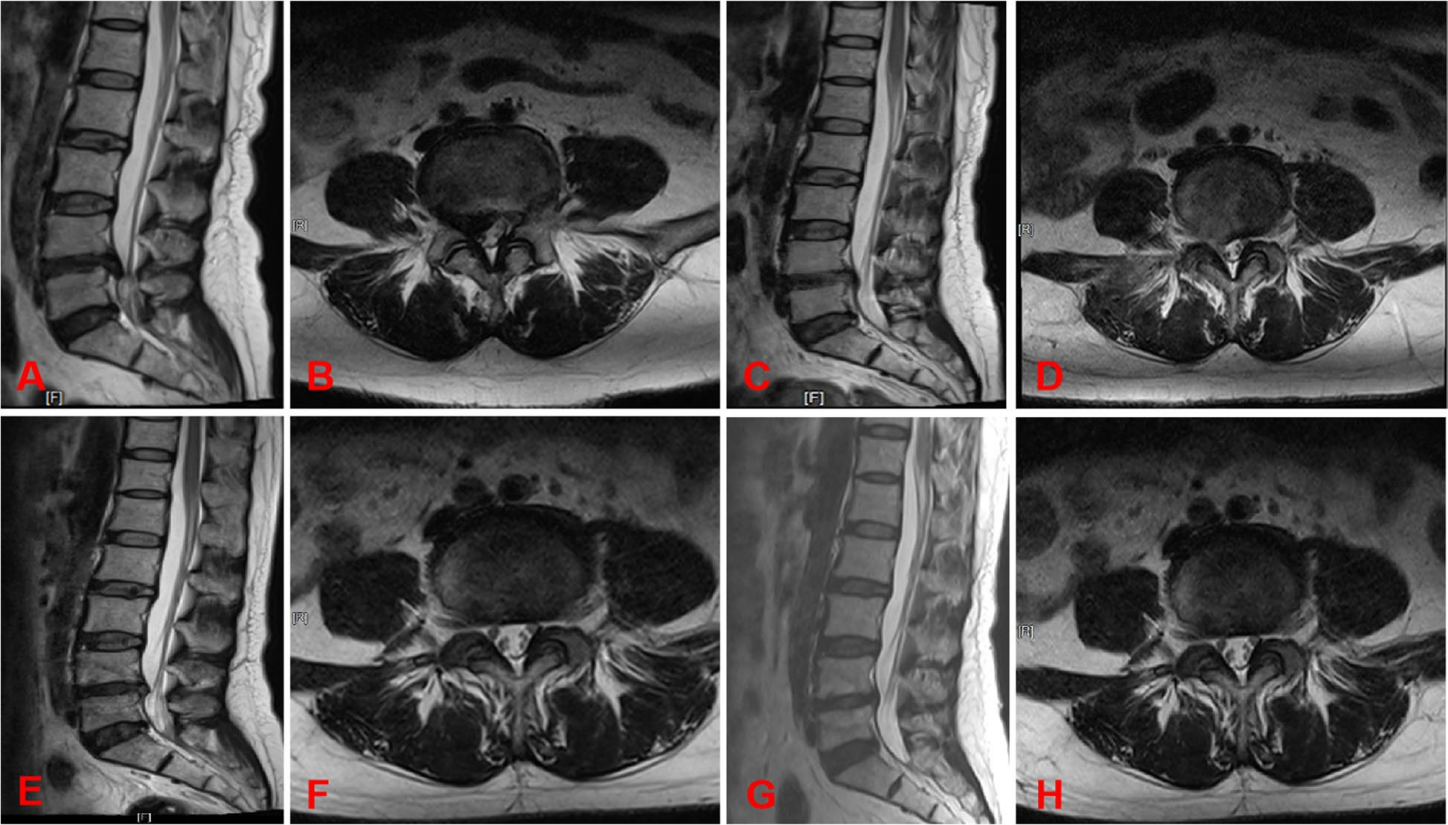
Aggressive discectomy. A-B Preoperative sagittal MRI and cross-sectional MRI; C-D sagittal MRI and cross-sectional MRI 3 days after surgery; E-F sagittal MRI and cross-sectional MRI 1 year after surgery;
